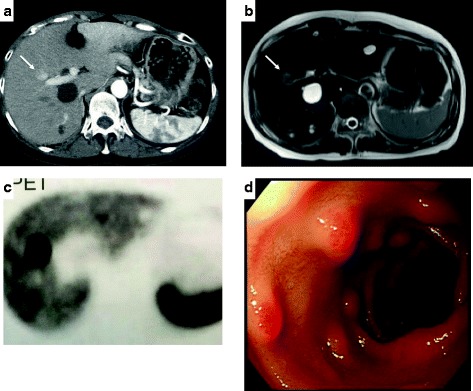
Preoperative imaging findings. a Abdominal contrast-enhanced computed tomography (CT) showing a hypervascular tumor in the posteroinferior segment of the right hepatic lobe (arrow). b Magnetic resonance imaging (MRI) showing a hypointensity tumor on T2-weighted imaging (arrow). c Somatostatin receptor scintigraphy (SRS) showing abnormal uptakes in the liver mass. d Upper gastrointestinal endoscopy showing several submucosal tumor-like lesions in the first to the second portion of the duodenum
